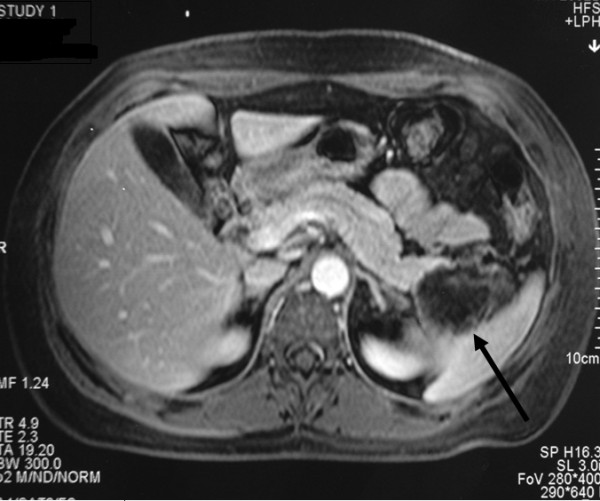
Magnetic resonance imaging (MRI) scan of the abdomen demonstrating a lesion of the pancreatic tail (arrow) with associated calcifications of the lesion's wall and a central cystic component.
